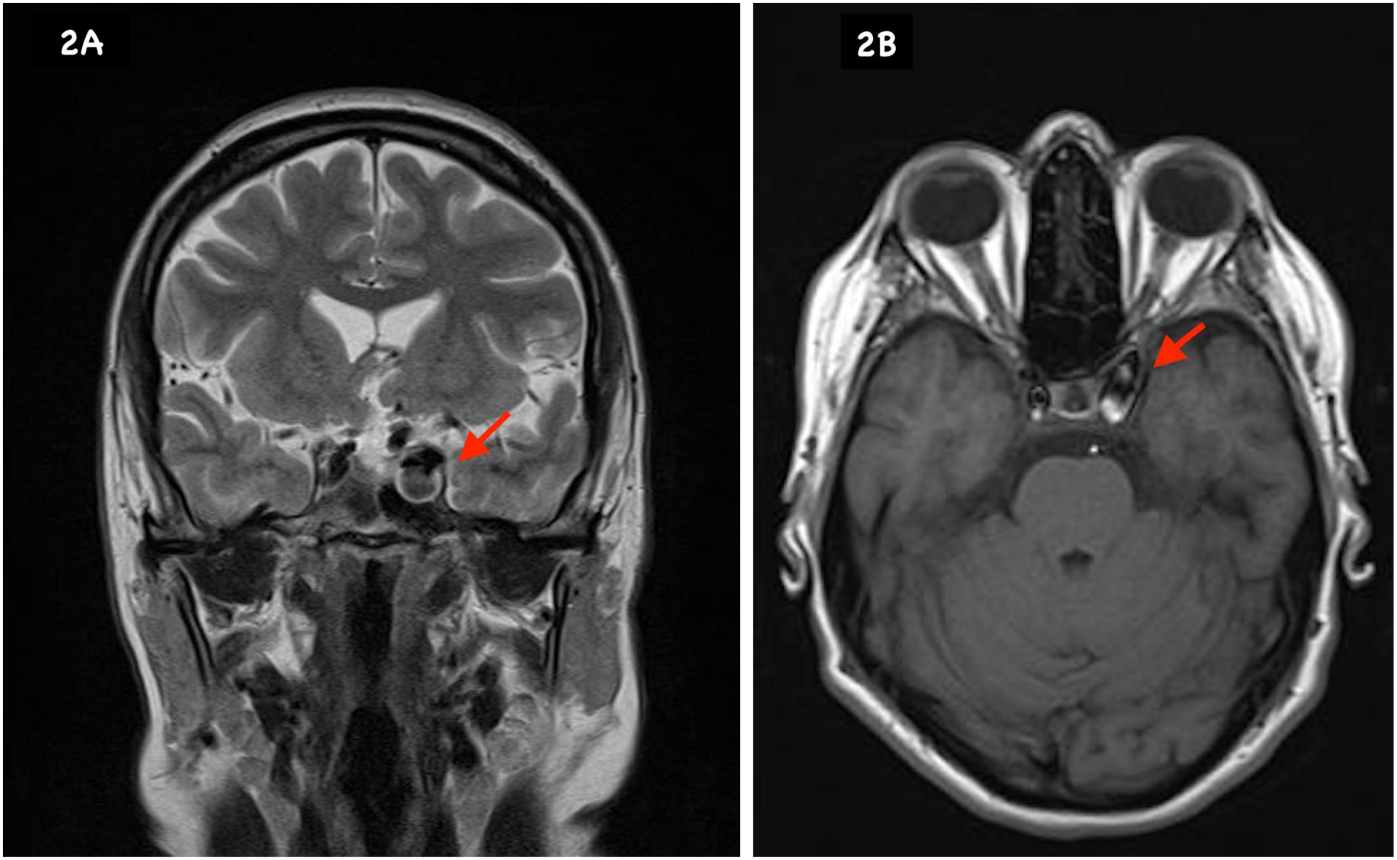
MRI T2 (A) and T1 (B) show a carotid-ophthalmic aneurysm.
